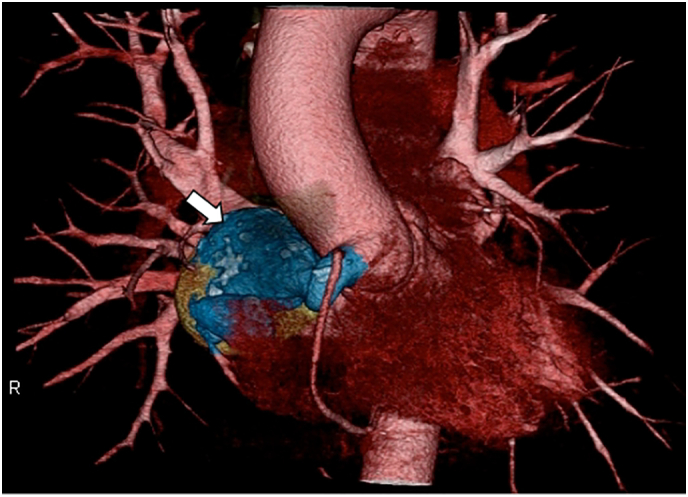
Preoperative computed tomography for the initial surgical procedure. An aneurysm measuring 53 × 48 mm is seen in proximity to the right aspect of the left atrium (white arrow), juxtaposed with the superior dorsal aspect of the right atrium and connected through a tubular structure originating from the sinus of Valsalva in the lower extremity of the ostium of the right coronary artery. Concomitantly, a fistulous communication is seen extending into the right atrium.
